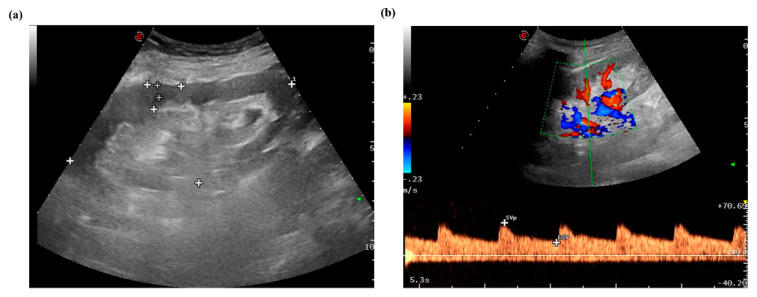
(a) B-mode image, measurement of renal size including renal length, width, and renal parenchyma thickness. The plus signs mean activating the measurement key after freezing the ultrasound imagine and the green color means focal position.(b) CDFI image, measurement of resistive index (RI).
